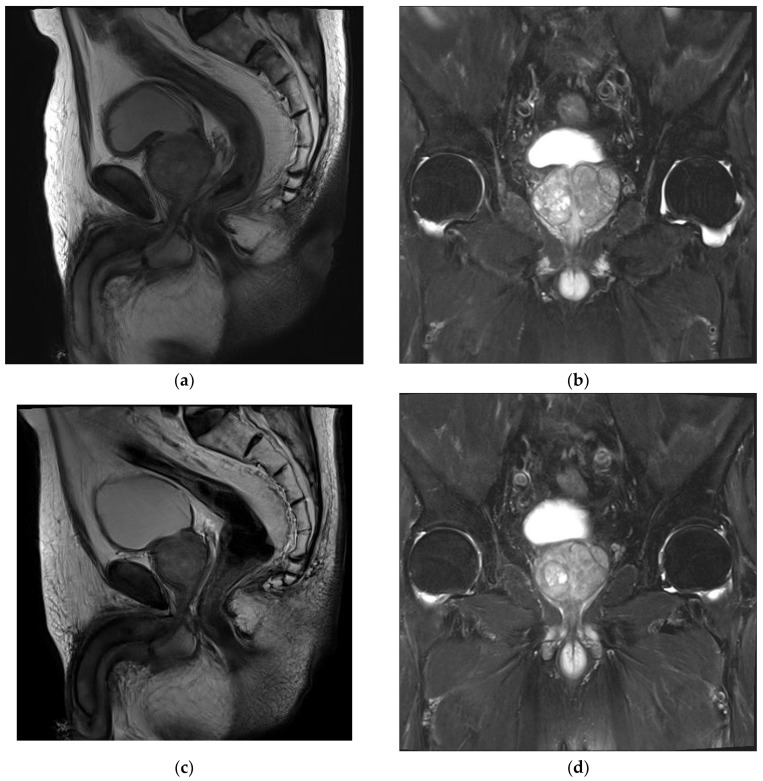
MRI images of patient’s BPH. (a,b) Pre-procedural MRI showed enlarged prostate of 89.39 cm^3^ (5.90 cm × 4.41 cm × 3.84 cm). (c,d) Post-procedural MRI at 24 months showed 66.05 cm^3^ (4.92 cm × 3.58 cm × 3.75 cm).
